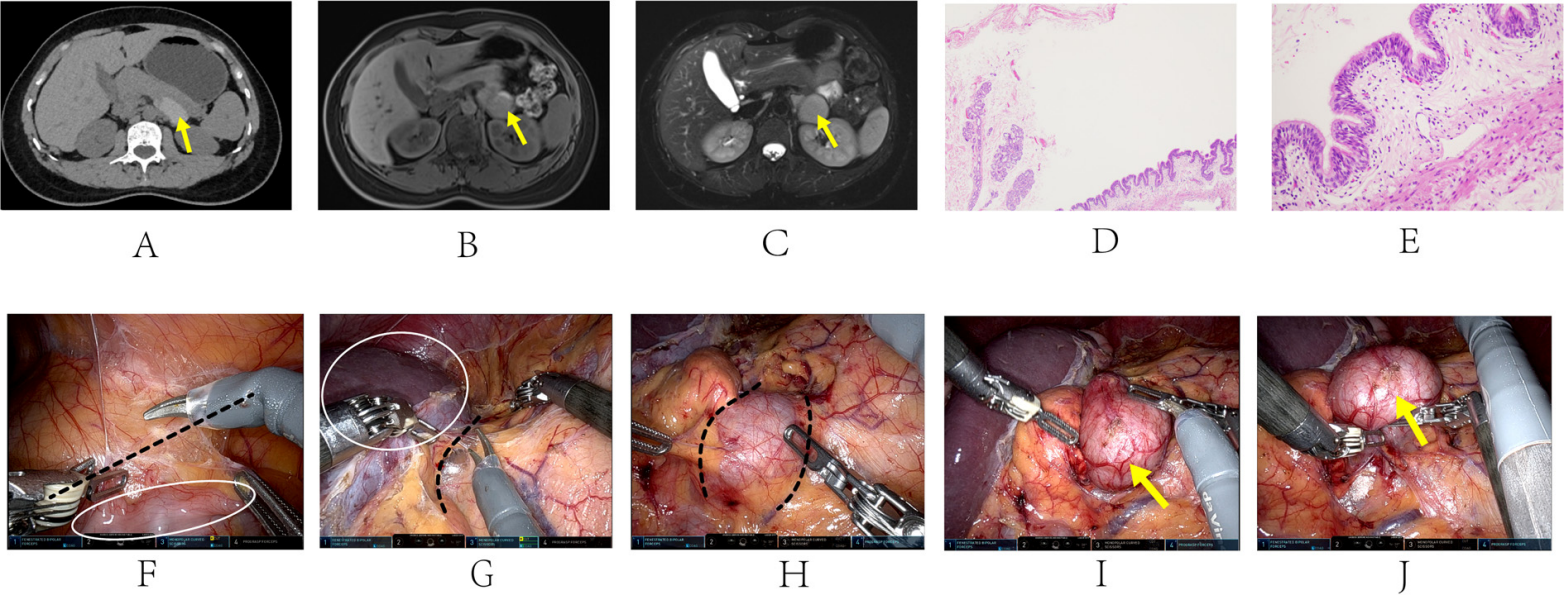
Radiological and pathological manifestations of Case 2 (A-E). Procedure of the robot-assisted surgery (F-J). The yellow arrow shows the location of the cyst: A: CT scan showed an ovoid, high-dense 4.2*1.9*4.3cm mass adjacent to abdominal aorta, with a HU value of 90; B: MRI image showed a 3.7*3.3*4.2cm cyst with a short signal at the T1-weighted stage; C: The cyst presented as a long signal on the MRI image at the T2-weighted stage, indicating the presence of mucinous cystic content. D: low-power magnification [40×]: Large cystic structures covered with characteristic ciliated columnar epithelium. E: high-power magnification [100×]: Mucous glands surrounded by smooth muscle tissue and cystic wall tissue. Fascia and soft tissue were separated along the black dashed line in image (F-H). (F) lift the lateral mesocolon of the descending colon, incise the left paracolic groove open. White circle: colon; (G) separate the anterior layer of the perirenal fascia and the plane of digestive system. White circle: spleen; (H, I) separate the tumor from the tail of the pancreas and the left perirenal fascia; (J) excise the cyst.
